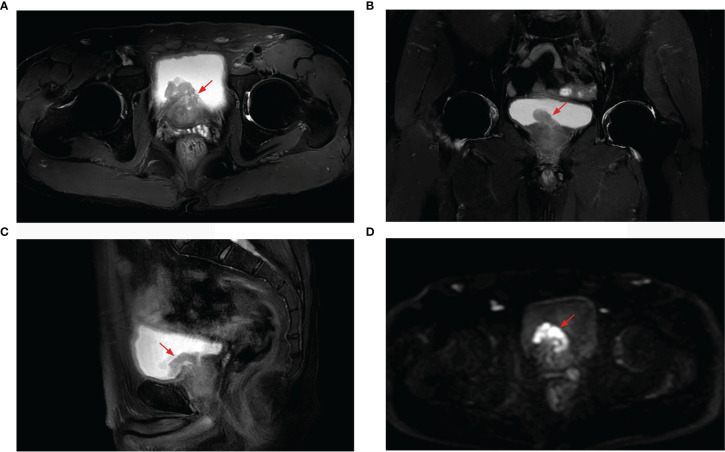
Prostate MRI revealing prostate cancer with bladder invasion, a marked hyperintensity on T2-weighted imaging [(A) axial, (B) coronal, (C) sagittal], and hypointensity on diffusion-weighted imaging (D).
